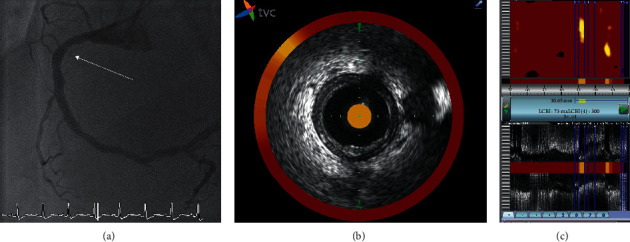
Example of an NIRS and IVUS investigation. (a) Angiogram of a nonculprit lesion located on the right coronary artery. The arrow shows the side branch, landmark for the IVUS analysis. (b) IVUS tomographic image of the right coronary artery obtained at the site of the side branch, showing a noncalcified atheroma. (c) NIRS chemogram from this lesion demonstrating the presence of a lipid-rich plaque (maxLCBI_4 mm_ measured at 300). IVUS = intravascular ultrasound; NIRS = near-infrared spectroscopy; and maxLCBI_4 mm_ = maximum lipid-core burden index in a 4 mm length of the artery.
